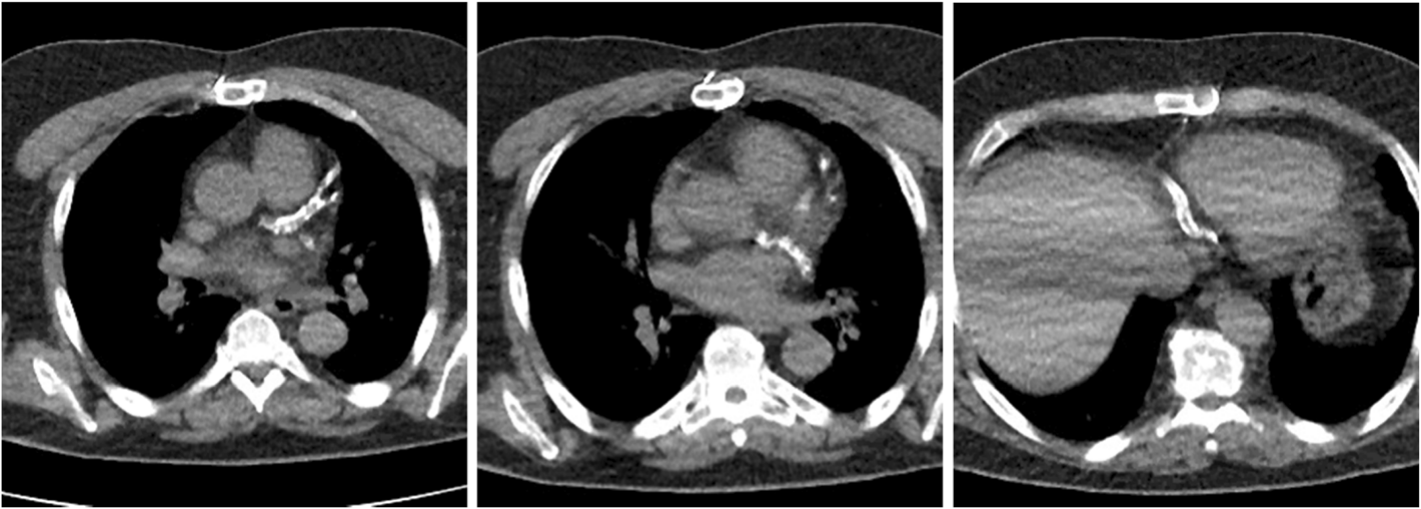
Axial CT images depicting extensive calcifation of the left anterior descending (*left*), left circumflex (*middle*), and distal right and posterior descending (*right*) coronary arteries
